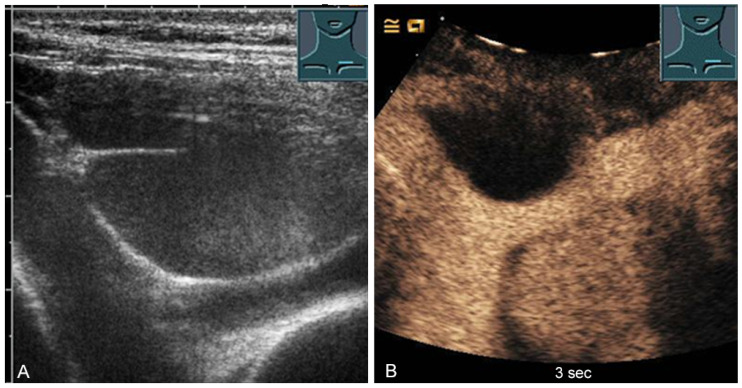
A cystic mediastinal mass on B-mode ultrasound (A) and contrast-enhanced ultrasound (B), confirmed mediastinoscopically as a regressive thymoma.
